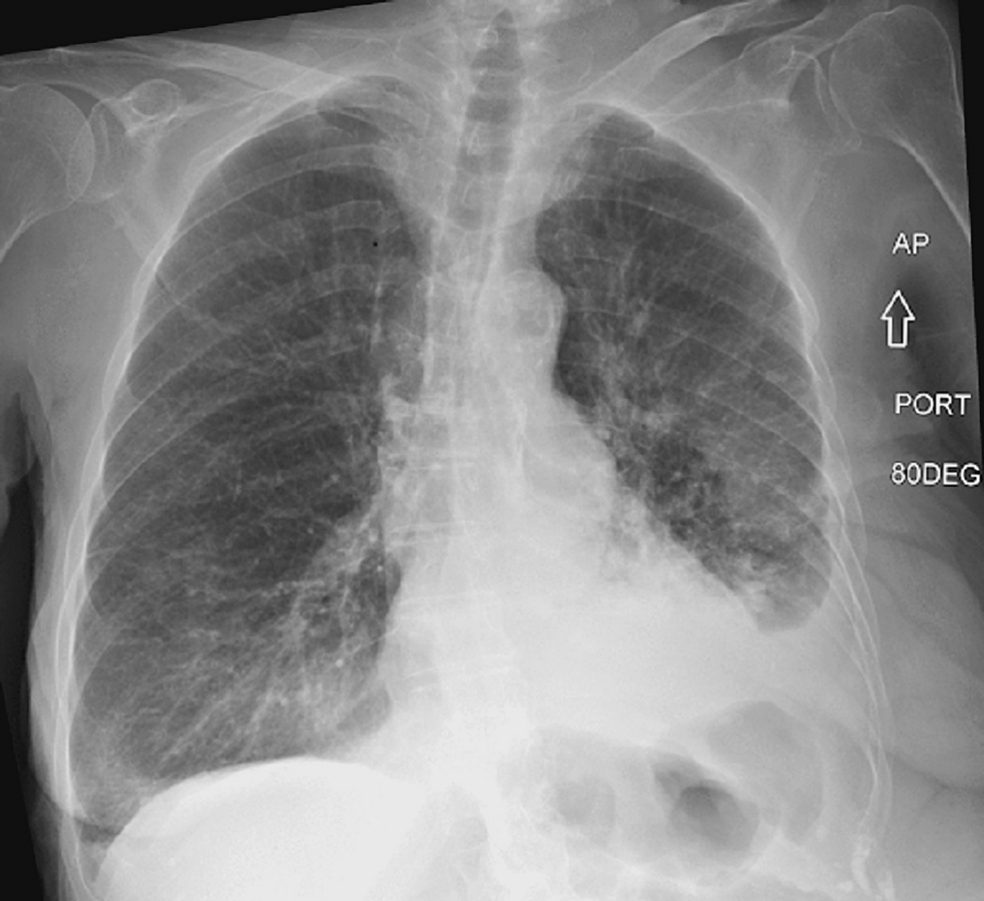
Chest x-ray showing haziness/infiltration on the left lung base along with left pleural effusion.
